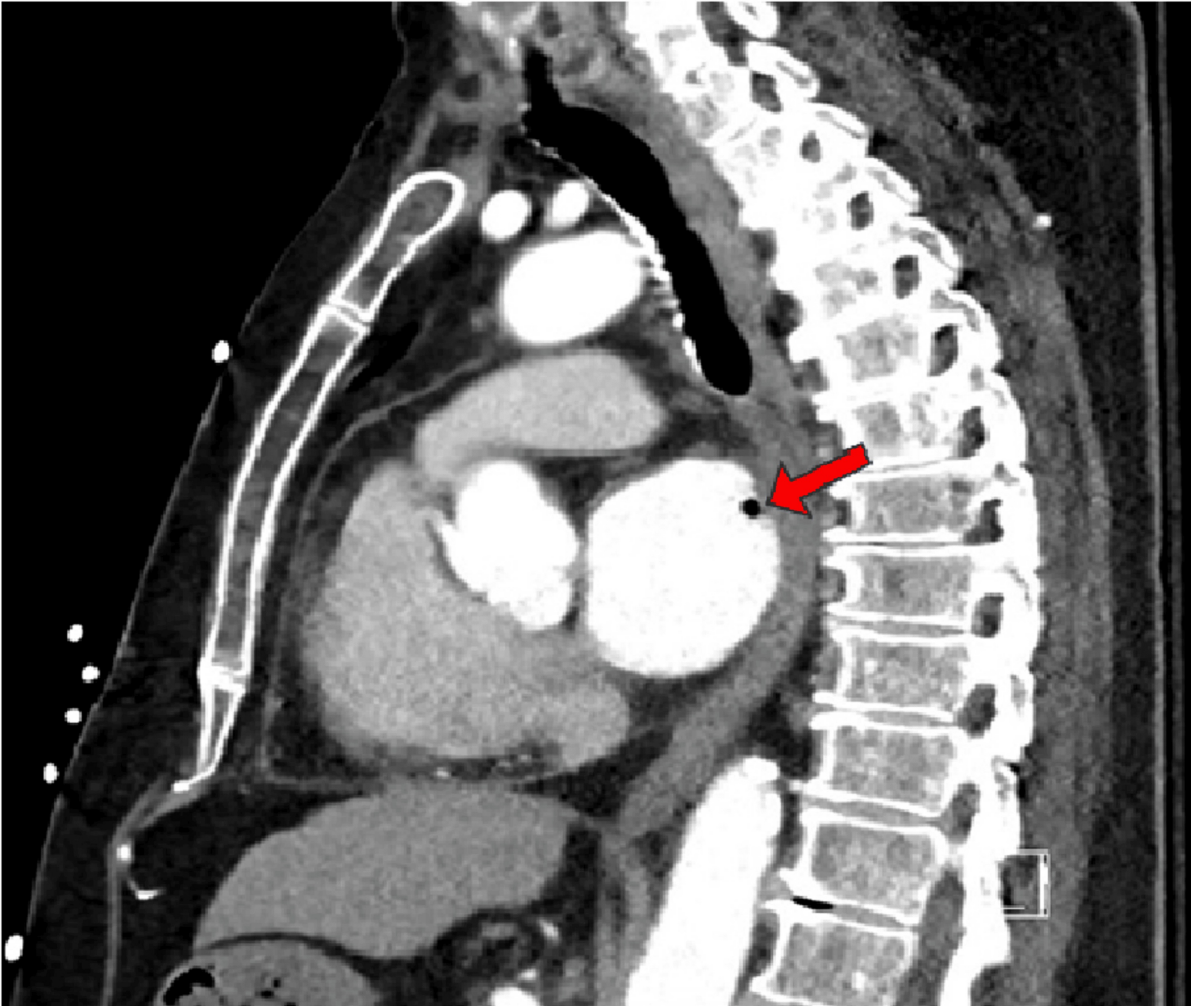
Sagittal view computed tomography (CT) of the thorax demonstrating the atrio-esophageal fistula (indicated by the red arrow)
